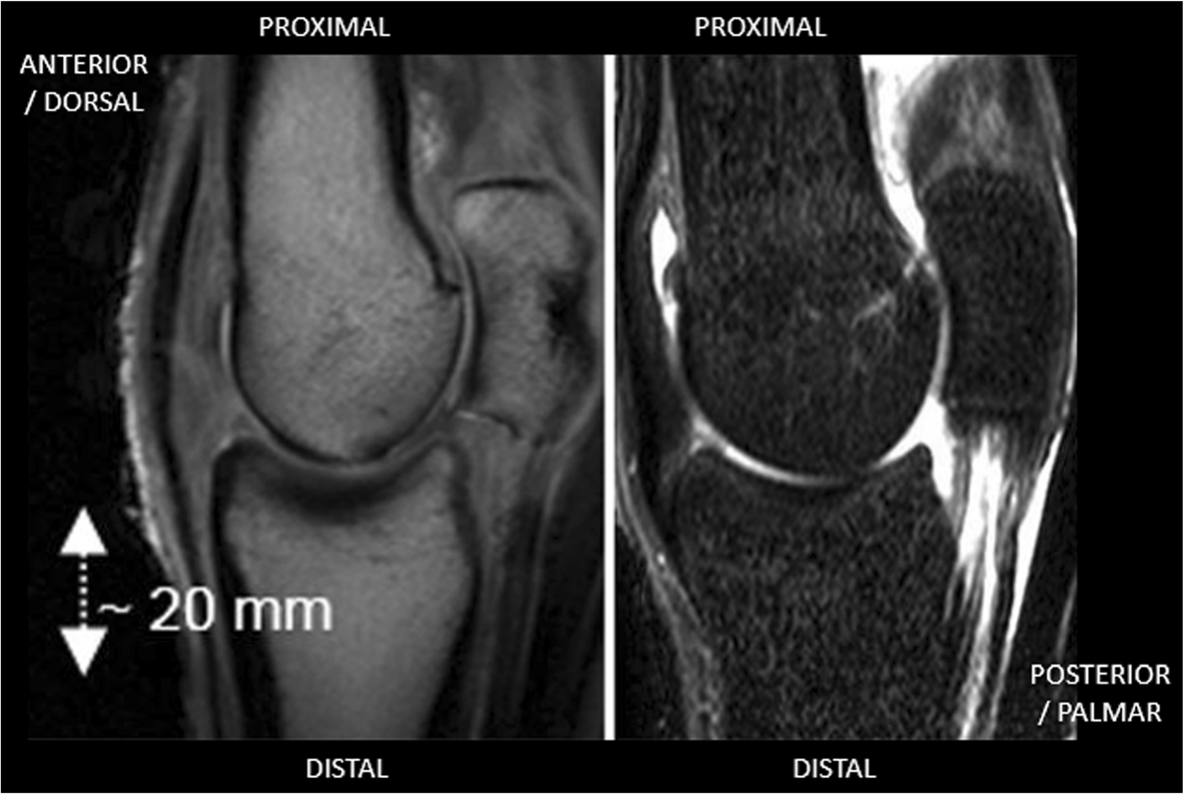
Parasagittal magnetic resonance images of the metacarpophalangeal joint. T1w image on left, STIR image on right, no evidence of BMOA
